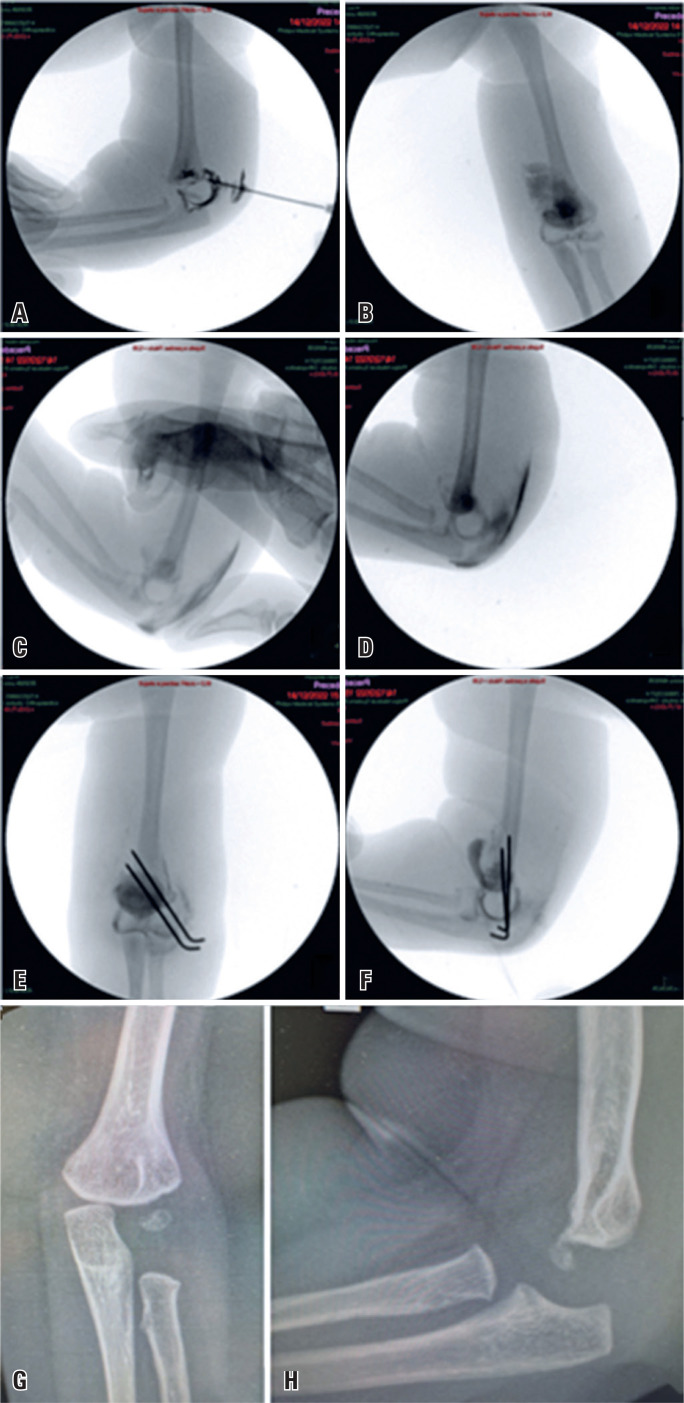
A and B) Assessment of the epiphyseal separation with the aid of an arthrogram; C) Successful closed reduction achieved with the elbow flexion maneuver and posterior support on the fragment; D) Loss of reduction upon elbow extension; E and F) Percutaneous fixation with two parallel lateral Kirschner wires; G and H) Radiographic images demonstrating consolidation and good alignment at 12 months after the injury
